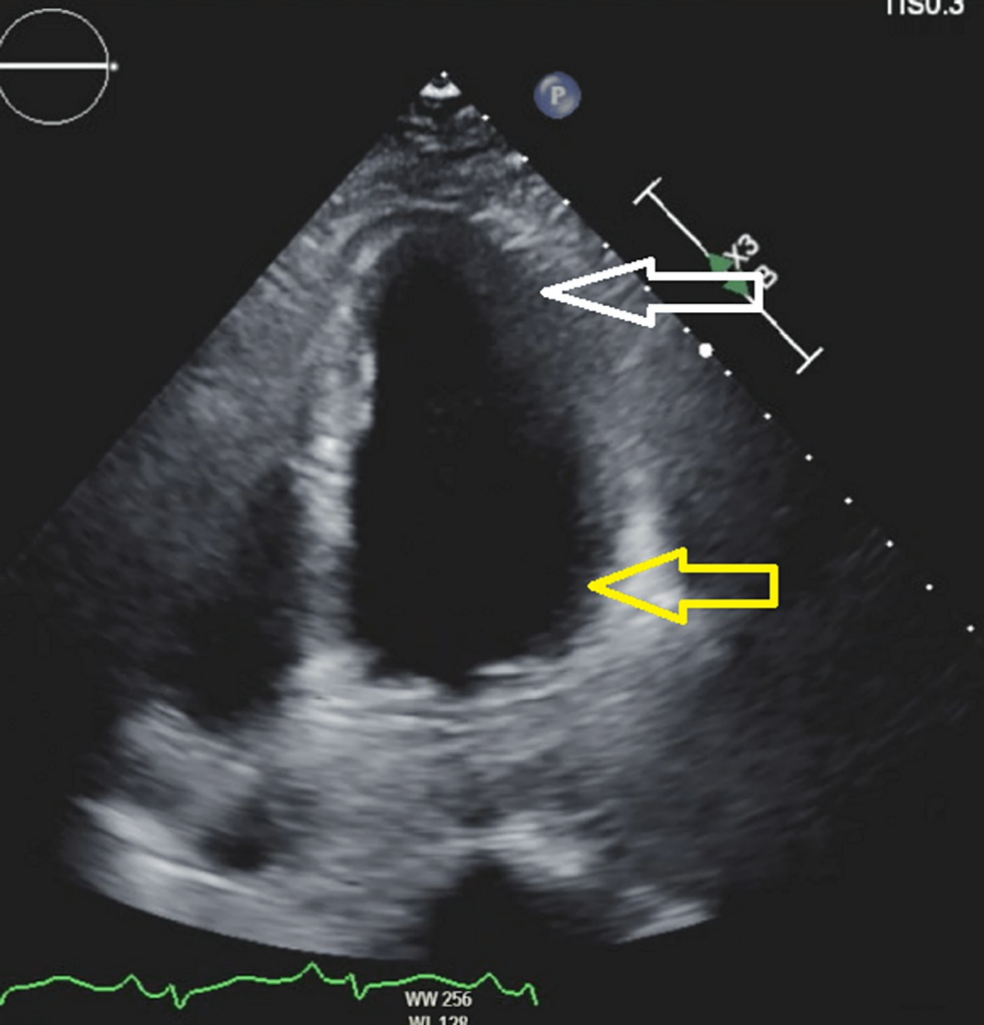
TTE (four-chamber view): apex (white arrow) and base (yellow arrow) of the LV chamber in diastolic relaxation TTE: transthoracic echocardiogram; LV: left ventricle
